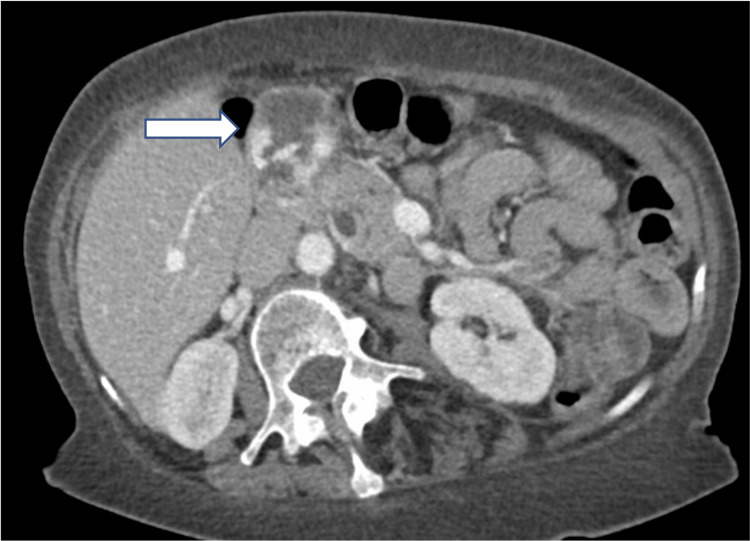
CT scan of abdomen showing duodenal diverticulum (arrow). On initial review by surgeons and radiologists the CT images were concerning for duodenal perforation with abscess.
